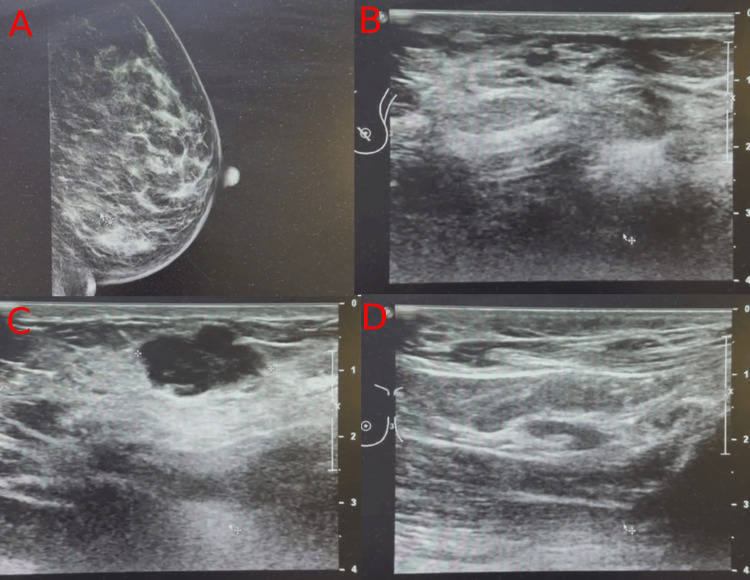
Follow-up mammogram (A) and ultrasound imaging (B-D).
